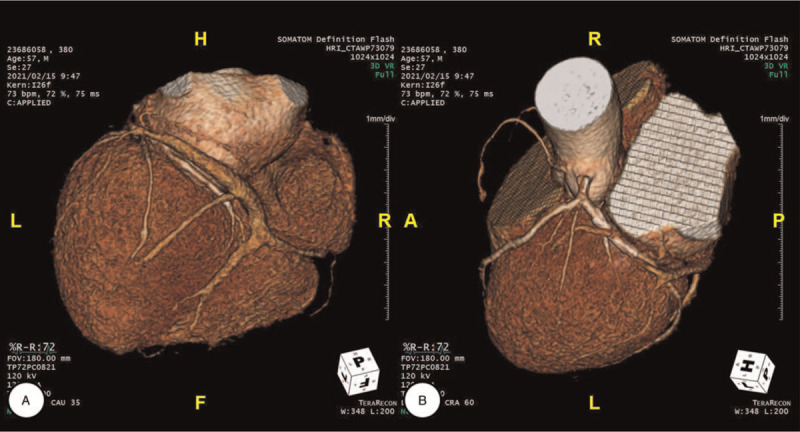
A, B, Coronary CTA demonstrates a normal LAD course, without calcified or noncalcified plaque formation. However, the ostium of the RCA is absent, except for only a small protruding branch from the LCC into the conus branch. Instead, a large branch originating from the LCX passes through the atrioventricular groove, supplying the RCA distribution. CTA = computed tomography angiography, LAD = left anterior descending coronary artery, LCC = left coronary cusp, LCX = left circumflex coronary artery, RCA = right coronary artery.
